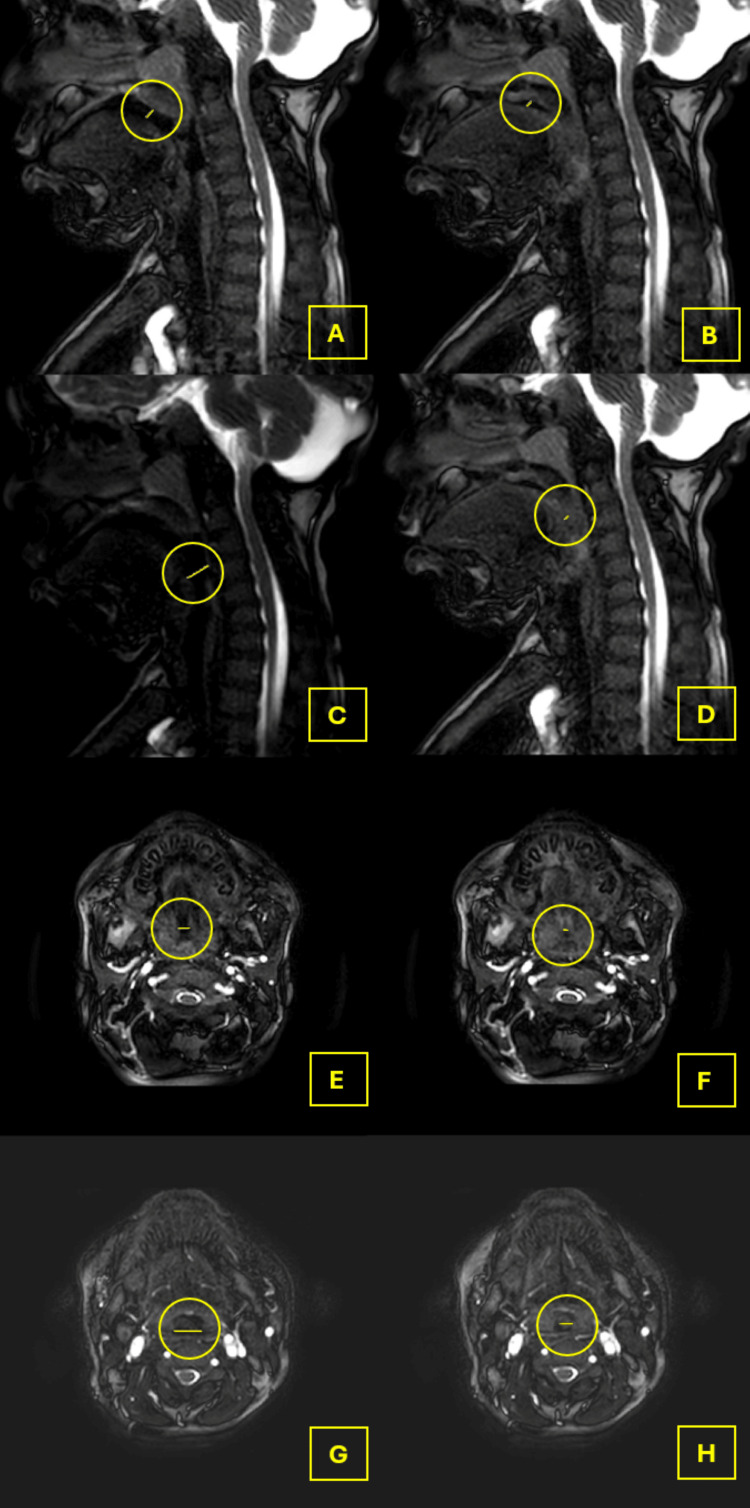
Measurements obtained in Cine-MRI A: A yellow line surrounded by a circle represents the maximal anteroposterior dimension of the nasopharyngeal level. B: A yellow line surrounded by a circle represents the minimal anteroposterior dimension of the nasopharyngeal level. C: A yellow line surrounded by a circle represents the maximal anteroposterior dimension of the oropharyngeal level. D: A yellow line surrounded by a circle represents the minimal anteroposterior dimension of the oropharyngeal level. E: A yellow line surrounded by a circle represents the maximal transverse dimension of the nasopharyngeal level. F: A yellow line surrounded by a circle represents the minimal transverse dimension of the nasopharyngeal level. G: A yellow line surrounded by a circle represents the maximal transverse dimension of the oropharyngeal level. H: A yellow line surrounded by a circle represents the minimal transverse dimension of the oropharyngeal level.
